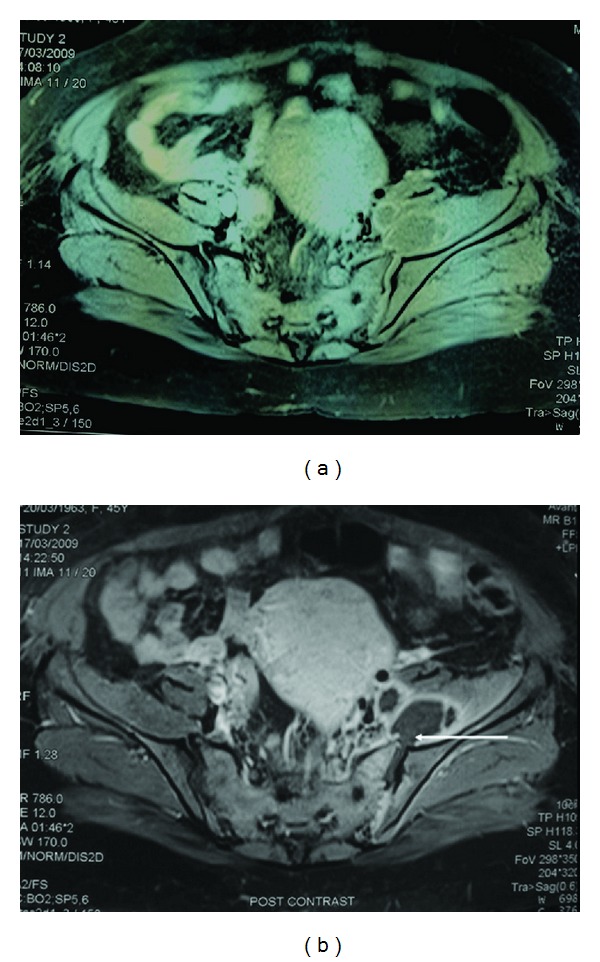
MRI of the pelvis and sacroiliac joints. Coronal T1-weighted (a) as well as (b) fat-saturated (FS) post IV contrast images of the pelvis and sacroiliac joints; showing a 5 cm in diameter multiloculated collection showing dense marginal enhancement deep to left iliopsoas muscle communicating with underlying left sacroiliac joint cavity (see arrow) with evidence of left sacroiliitis in the form of irregular articular surface with subarticular thickening as well as enhancement.
